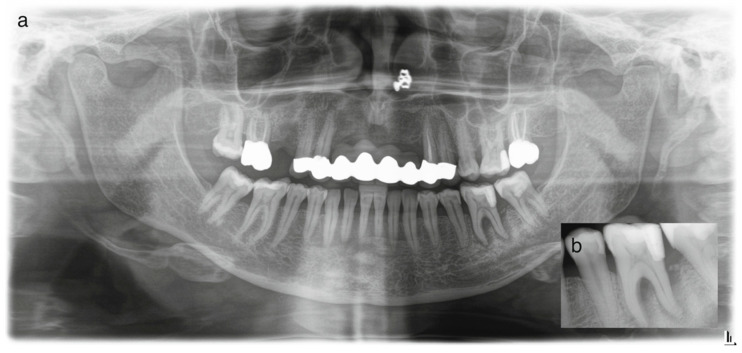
Panoramic radiograph at the initial visit (a); periapical radiograph at the initial visit displaying extensive periradicular radiolucency with evidence of bifurcation involvement (b).
